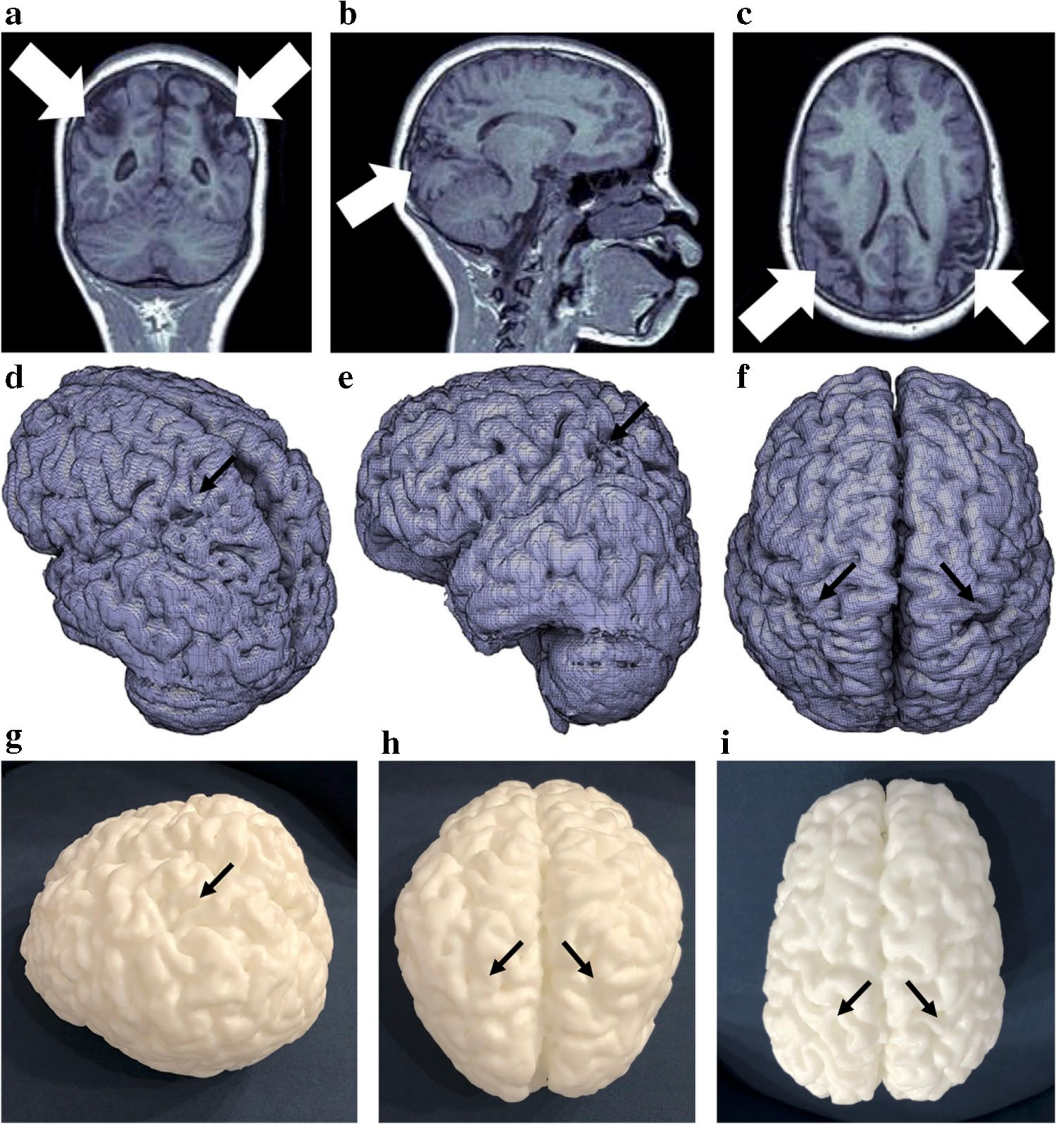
A 10-year-and-11-month-old girl who suffered a perinatal hypoxic ischemic injury with a watershed pattern of injury. a–c Coronal (a), sagittal (b) and axial (c) T1W magnetic resonance images show bilateral and symmetric cortical and subcortical atrophy in the posterior and perisylvian watershed regions (*arrows*). d–f Oblique (d), lateral (e) and vertex (f) views of the 3-dimensional (D) mesh virtual model with the corresponding areas of posterior and perisylvian watershed atrophy seen as prominence of the surface sulci with decreased size of the gyri (*arrows*). g–i Oblique (g), posterior vertex (h) and center vertex (i) photographic views of the corresponding 3-D print models show the affected areas (*arrows*)
